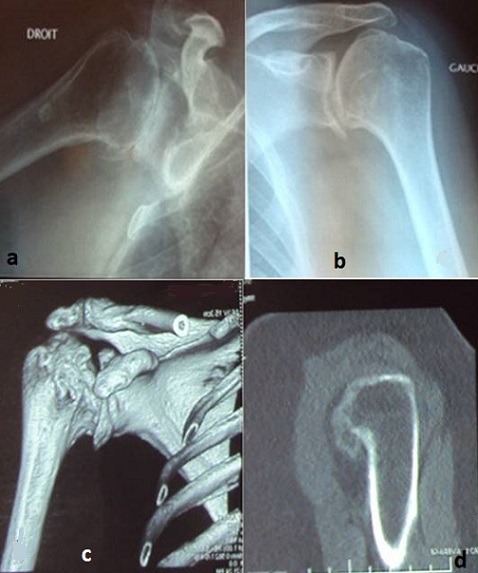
(a,b) la radiographie standard numérique des deux épaules a objectivé une omarthrose avancée de l'articulation glèno-humérale, classé IV; (c,d) la TDM des deux épaules avec reconstruction bidimensionnel (2D) et tridimensionnel (3D)
